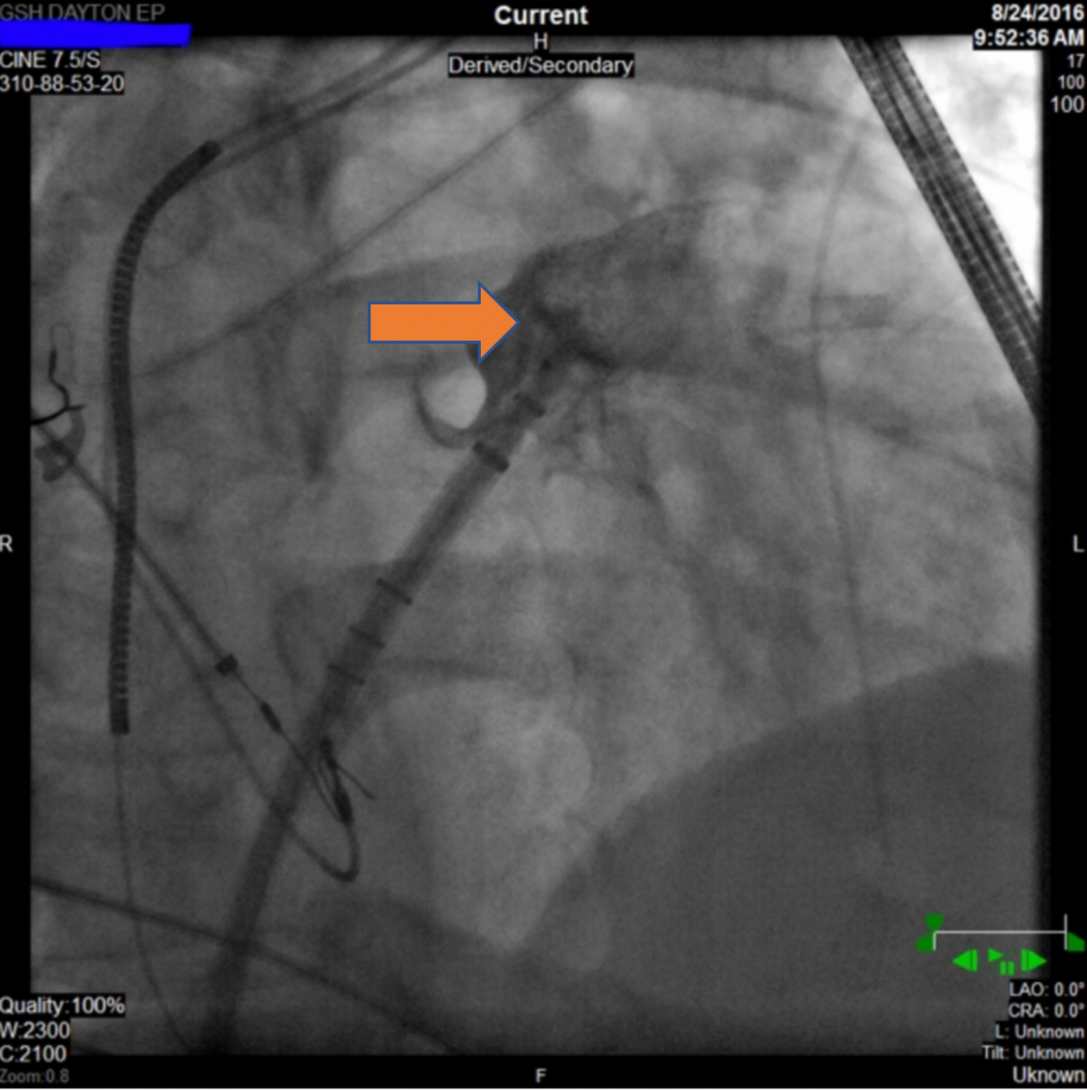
Fluoroscopic image while deploying the left atrial occlusion device Watchman (red arrow). TEE, transesophageal echocardiogram
